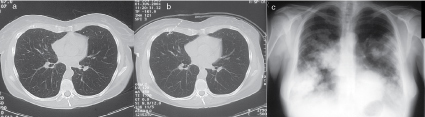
Chest CT image from June 2003 (a) shows no pathological findings in the anterior part of the middle lobe of the right lung. Chest CT scan from June 2004 (b) of the same location, as in Figure 2a, shows a subpleural, spiculated nodule (arrow) in the anterior part of the right lung middle lobe. The chest radiograph from August 2006 (c) shows multiple lung metastases from chondrosarcoma.
